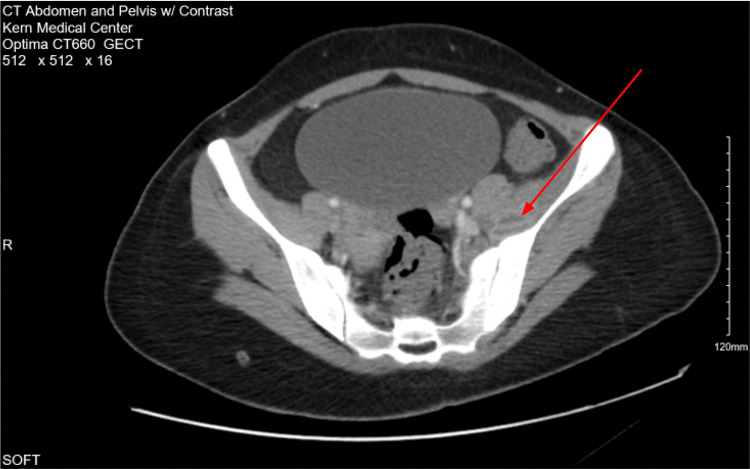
Axial contrast-enhanced CT scan of the abdomen and pelvis demonstrating a left-sided psoas abscess (arrow) CT: computed tomography
